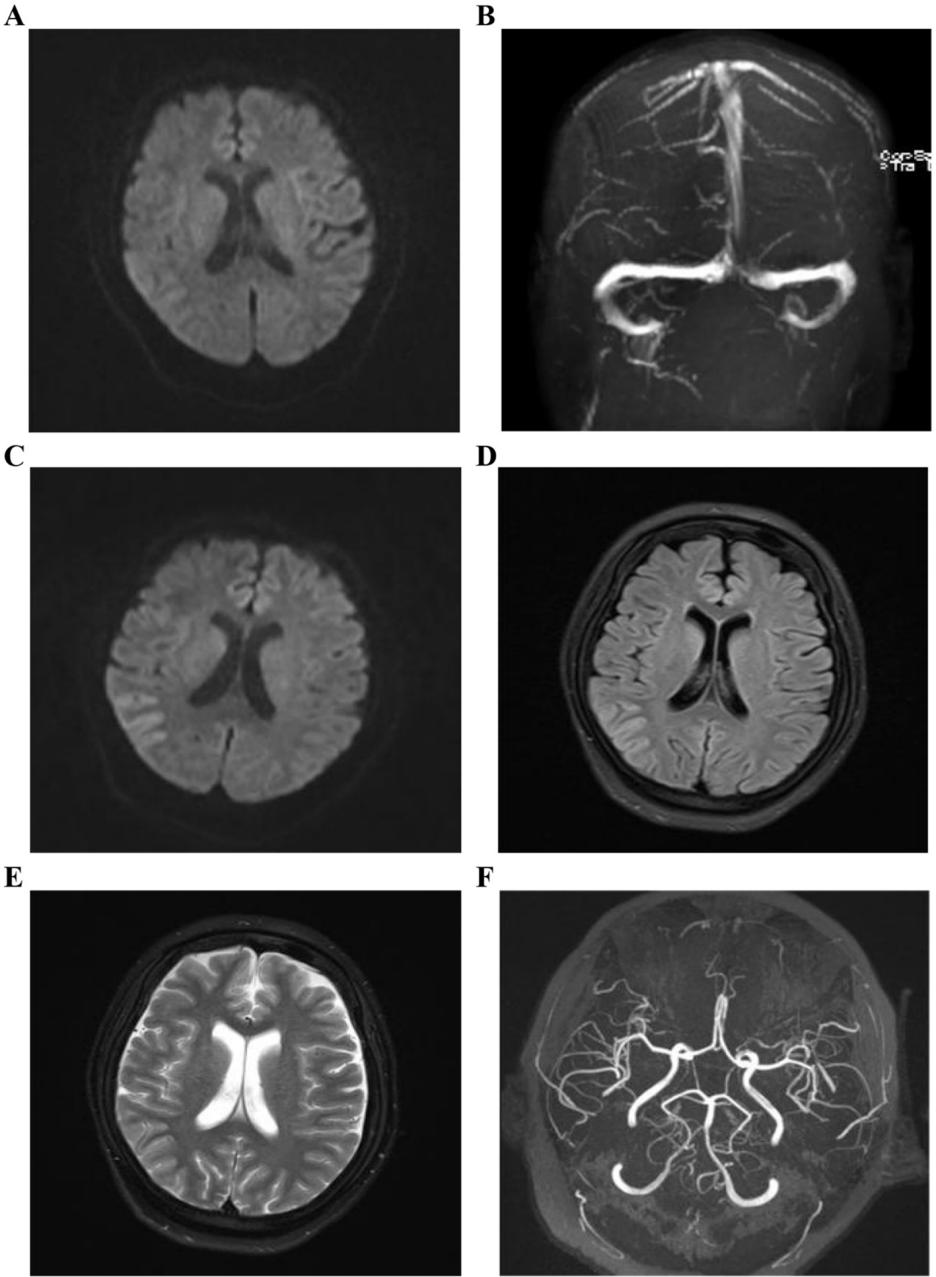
Magnetic resonance images of the patient after the onset of symptoms. (A, B) DWI and MRV showed no abnormalities on the ninth day of illness. DWI, diffusion weighted imaging; MRV, monitoring reporting verification; (C-F) MRI and MRA taken on day 14 of illness showed mild swelling of the right temporoparietal cortex with DWI hyperintensity and normal vascular findings, respectively. MRI, magnetic resonance imaging; MRA, magnetic resonance angiography; DWI, diffusion weighted imaging.
